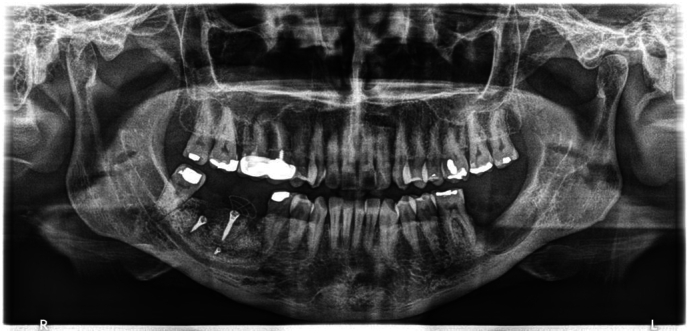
Panoramic x-ray follow-up.
